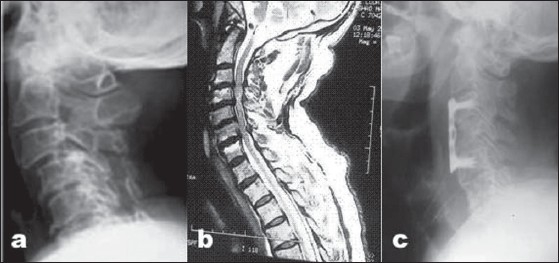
(a) X-rays cervical spine (lateral view) showing cervical spondylotic myelopathy (b) T2W MRI (saggital section) showing cervical spondylotic myelopathy post-operative (c) Post operative X-ray cervical spine showing corpectomy and anterior cervical plate
